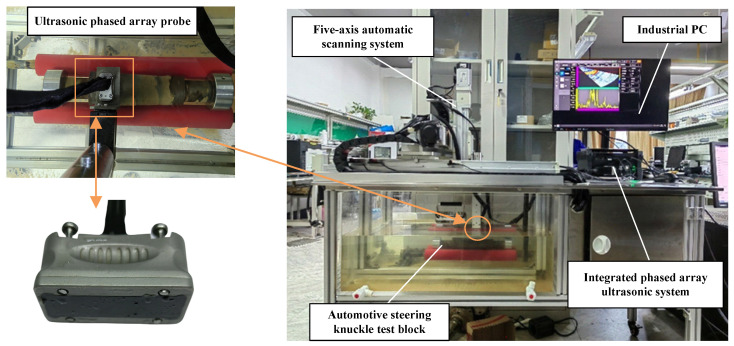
Water-immersion phased array ultrasonic testing system.
